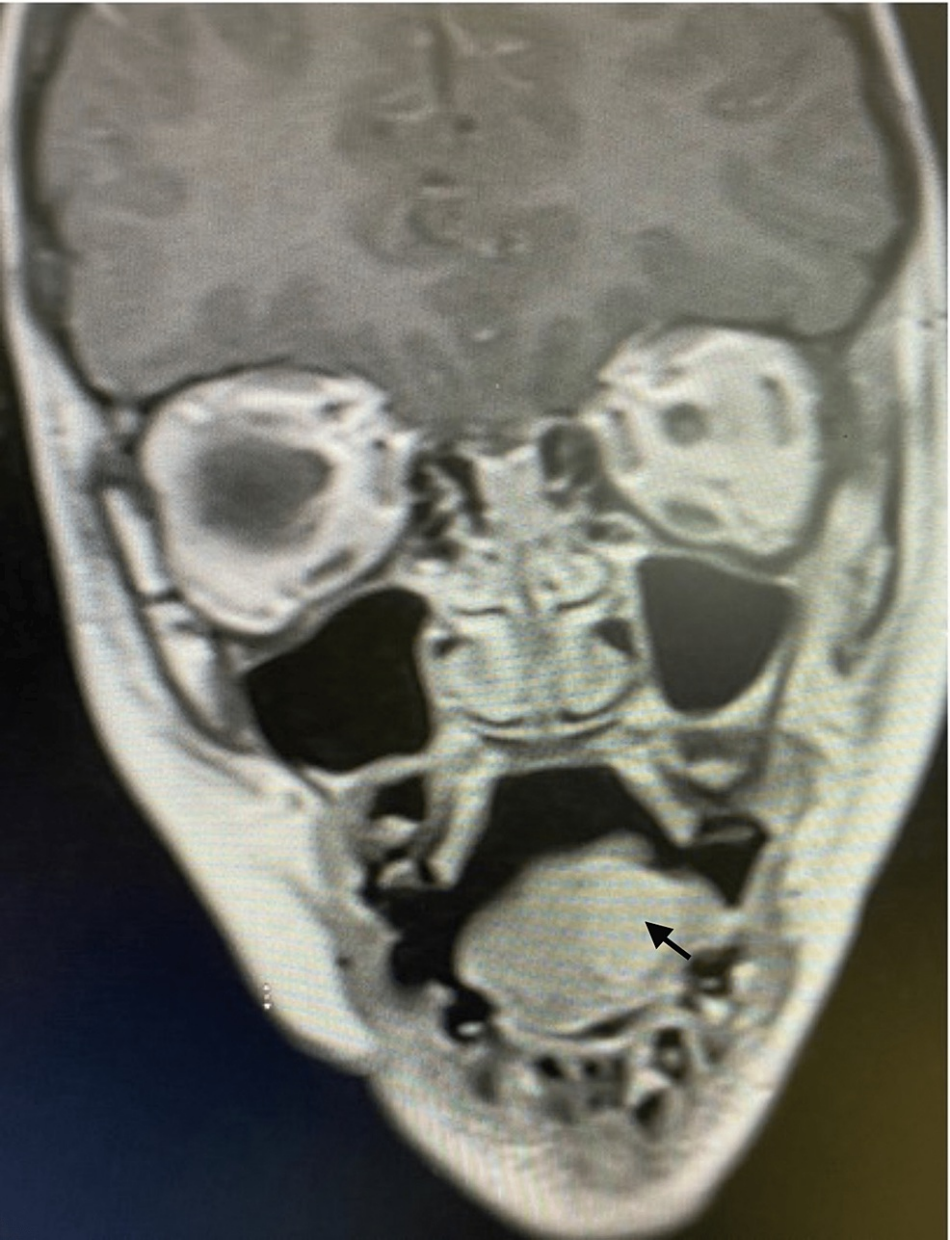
Coronal T1 post-contrast magnetic resonance imaging (MRI) of the head demonstrates the cranial caudal dimension of the enhancing lesion (black arrow) measuring 1.7 cm with no surrounding edema or regional lymphadenopathy.
